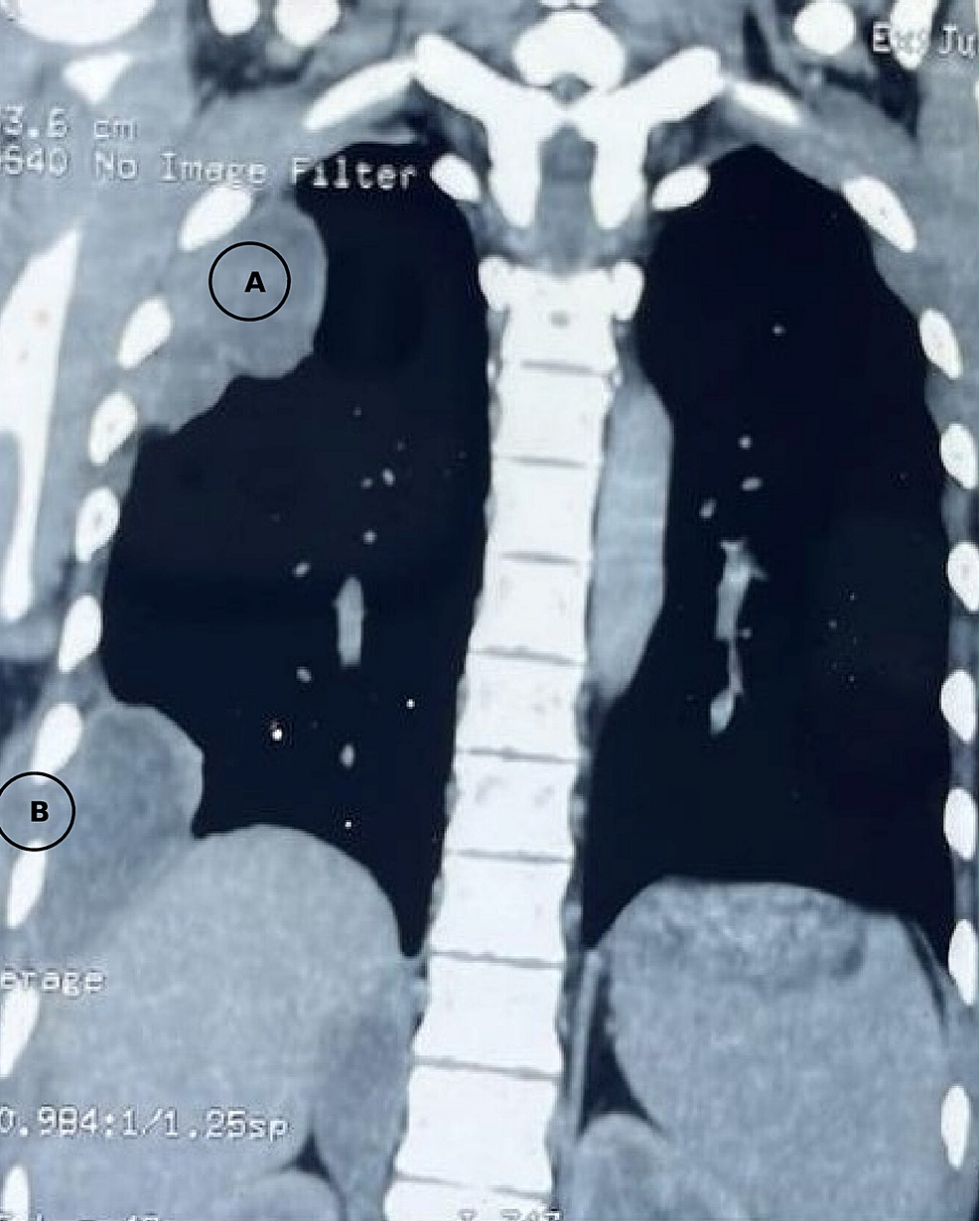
Contrast-enhanced computed tomography (CECT) thorax showing loculated pleural effusion (A) and pleural effusion with communication to subcutaneous plane (B).
